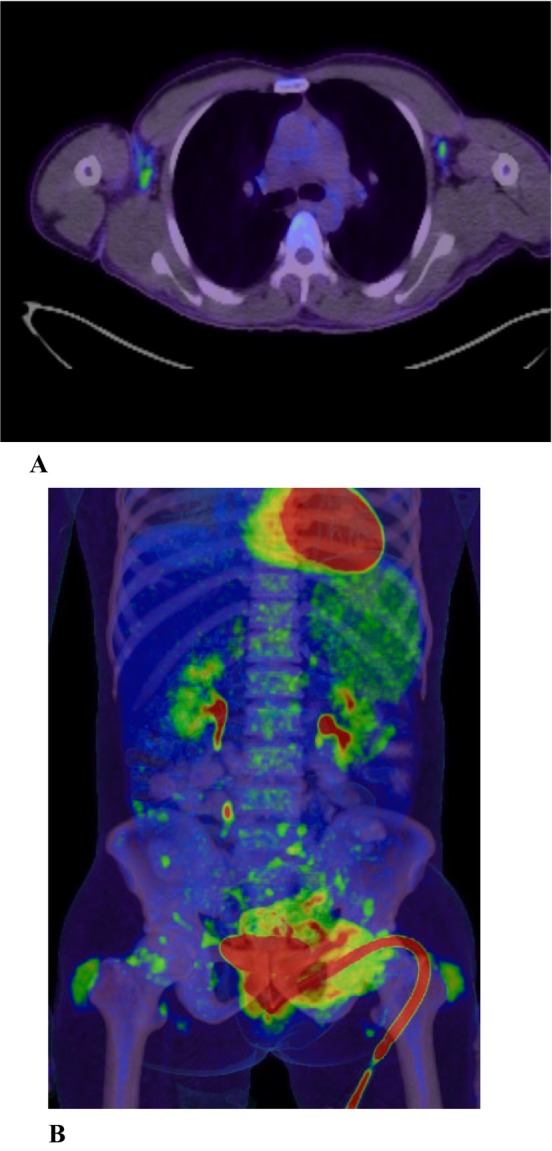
(A) PET CT showing slightly enlarged moderately hypermetabolic lymph nodes are seen in both axillary regions. (B) CT showing Hepatosplenomegaly. Hyperactive spleen and bone marrow. Mildly enlarged lymphadenopathy in the abdomen. Overall activity in these lymph nodes is of mild degree. The findings may indicate a low‐grade lymphoma with differential diagnosis of systemic infection.
